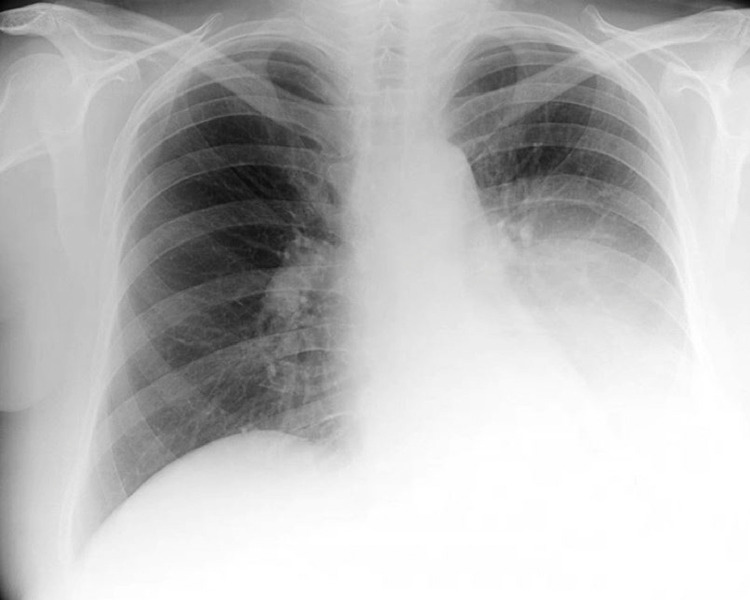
Chest X-ray at presentation; total opacification of the left lower lobe.
